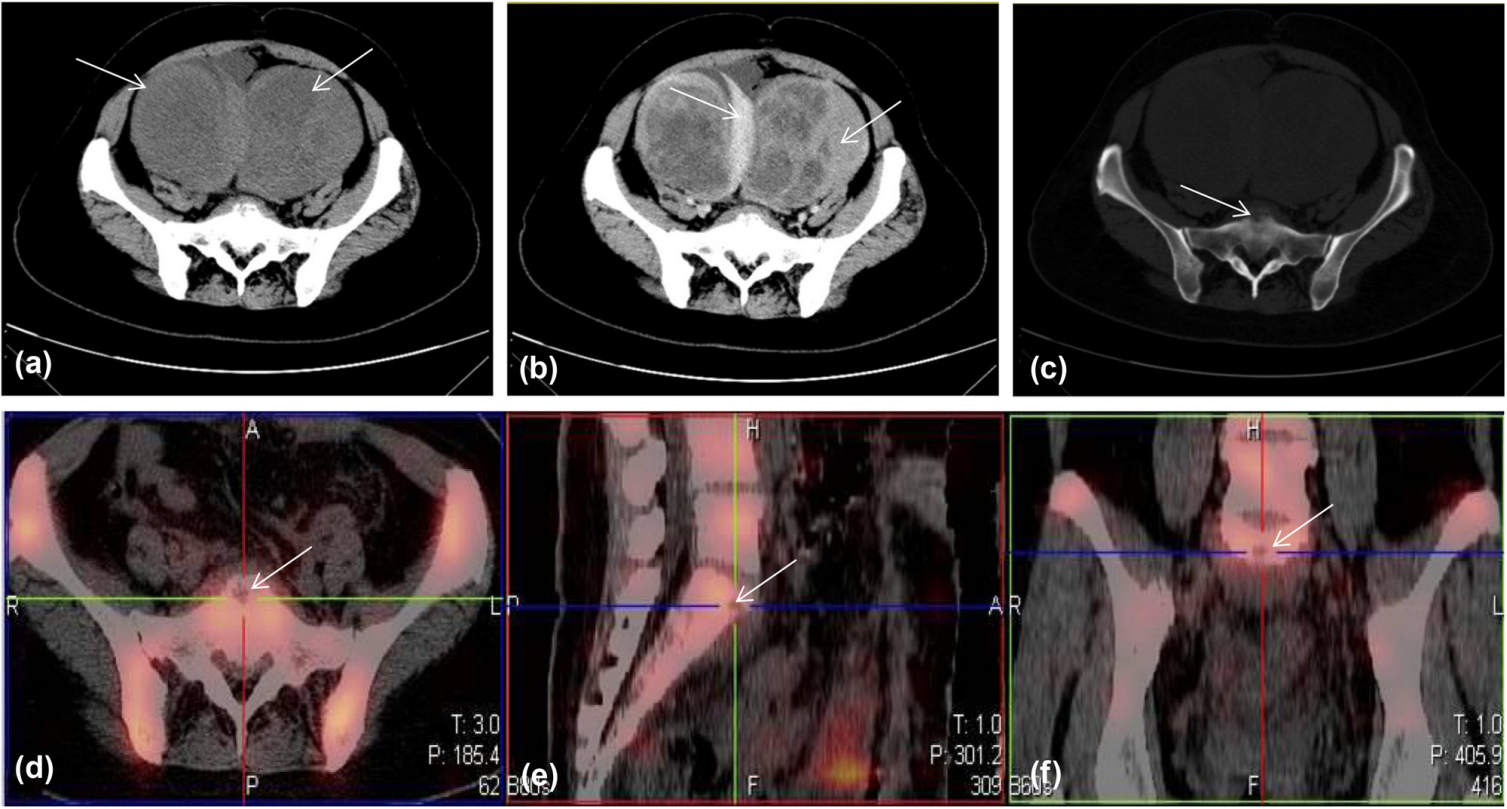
Whole abdomen CT (a–c) and radionuclide bone scintigraphy (d–f). (a) Plain abdominal CT scan. Arrows indicate two enlarged ovarian tumors with uneven density at two sides of the uterus. (b) Enhancement phase of full abdominal CT. The boundary and solid portion of the two ovarian tumors are enhanced in the arterial phase. (c) Bone window showed a discontinuous border and soft tissue density mass in front of the sacral promontory, indicating lesion invasion. (d–f) Radionuclide bone scintigraphy also showed focal radionuclide concentration of the first sacrum at transverse and median sagittal and coronal sections.
